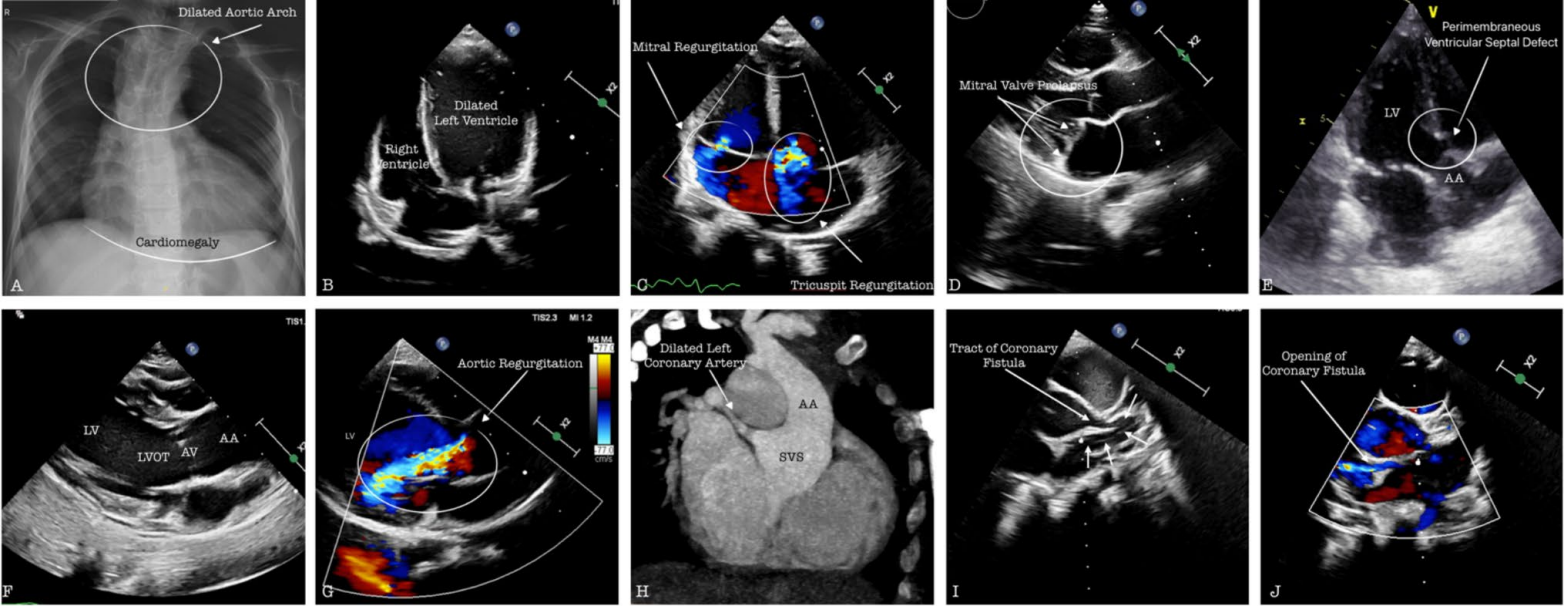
Collage of some screenshots from imaging methods. A Posterior-anterior view of chest X-ray: dilated aortic arch and cardiomegaly are noted. B Apical 4-chamber echocardiography image, left ventricular dilatation is seen. C–D Apical 4-chamber and long-axis echocardiography image: mitral valve prolapse and atrioventricular valve regurgitation are observed with color Doppler USG. E 2D apical 5-chamber echocardiography image, ventricular septal defect closed by spontaneous tricuspid pouch is observed. F–G Long-axis image shows left ventricle (LV), left ventricular outflow tract (LVOT), aortic valve (AV), dilated ascending aorta (AA), and aortic regurgitation. H–J 3D computed tomography and 2D short-axis echocardiographic images show a dilated sinus valsa (SVS) and ascending aorta (AA), coronary-cameral fistula originating from the left coronary artery and opening into the right atrium
